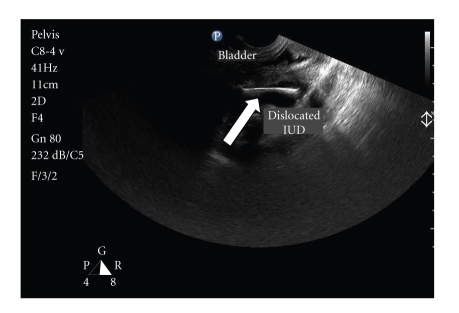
Transvaginal sonography showed a misplaced intrauterine device (arrow) that seemed to be located in the uterovesical space and embedded in the left posterior wall of the bladder.
